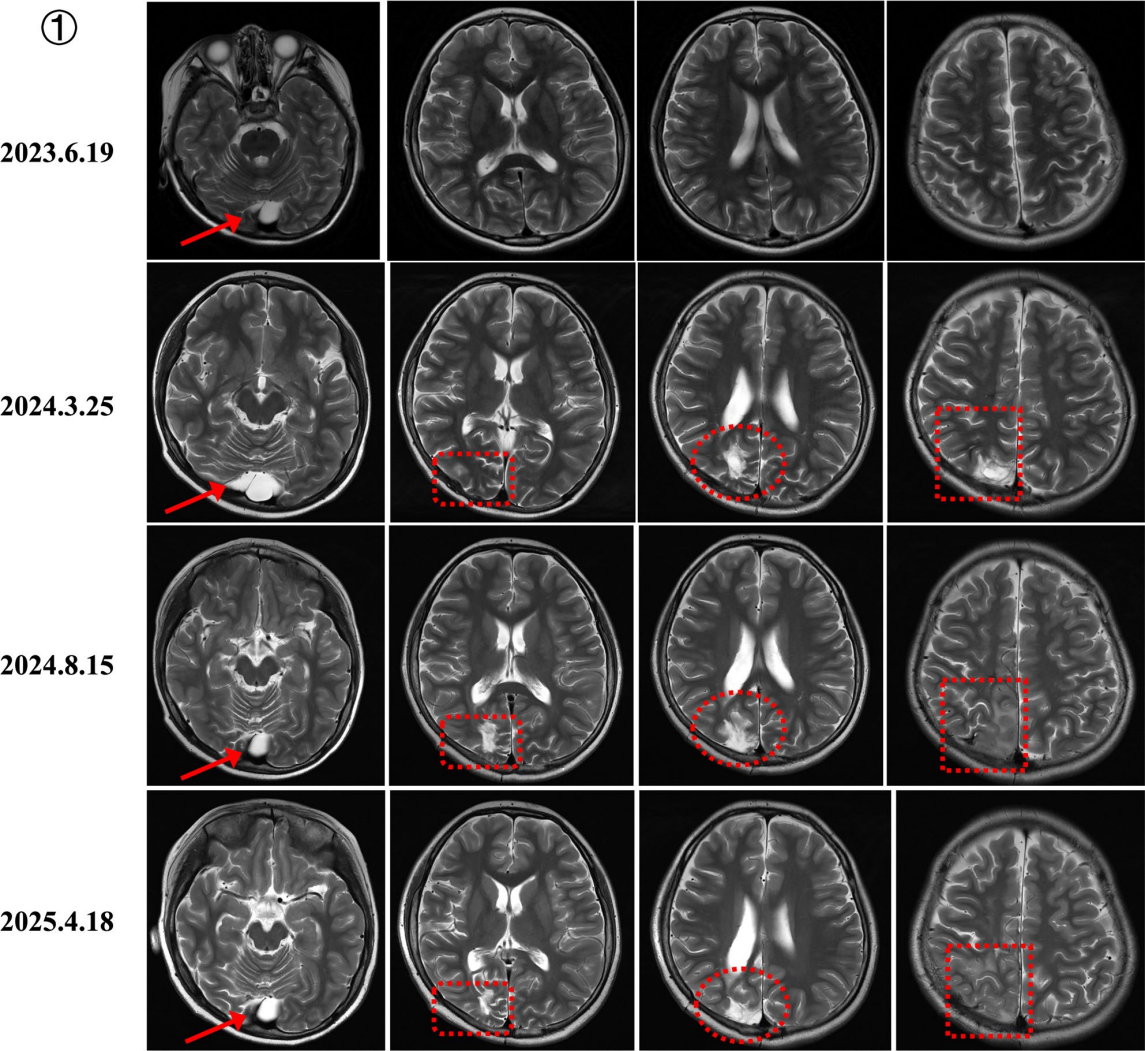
Serial brain MRI in a MELAS patient with MT-TL1 m.3243 A > G mutation (2023–2025). Progressive cortical involvement is shown, correlating with refractory seizures and motor decline. Improvement was temporarily achieved after SEEG-guided thermocoagulation (July 2023) and subtotal lobectomy (January 2024), followed by recurrence and further progression in the right hemisphere (April 2025)
